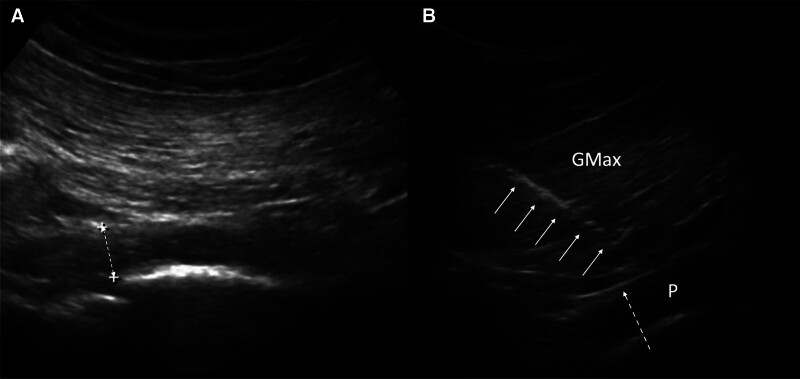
Muscle identification and injection. (A) The gluteus maximus and piriformis muscle were identified using a curved probe. The thickness of the piriformis muscle was measured using ultrasound (dotted 2-way arrow). (B) An ultrasound-guided injection was performed, targeting the piriformis muscle and fascia between the gluteus maximus and piriformis muscle. White arrows indicate the needle, and dotted arrows indicate fascia. GMax = gluteus maximus muscle, P = piriformis muscle.
